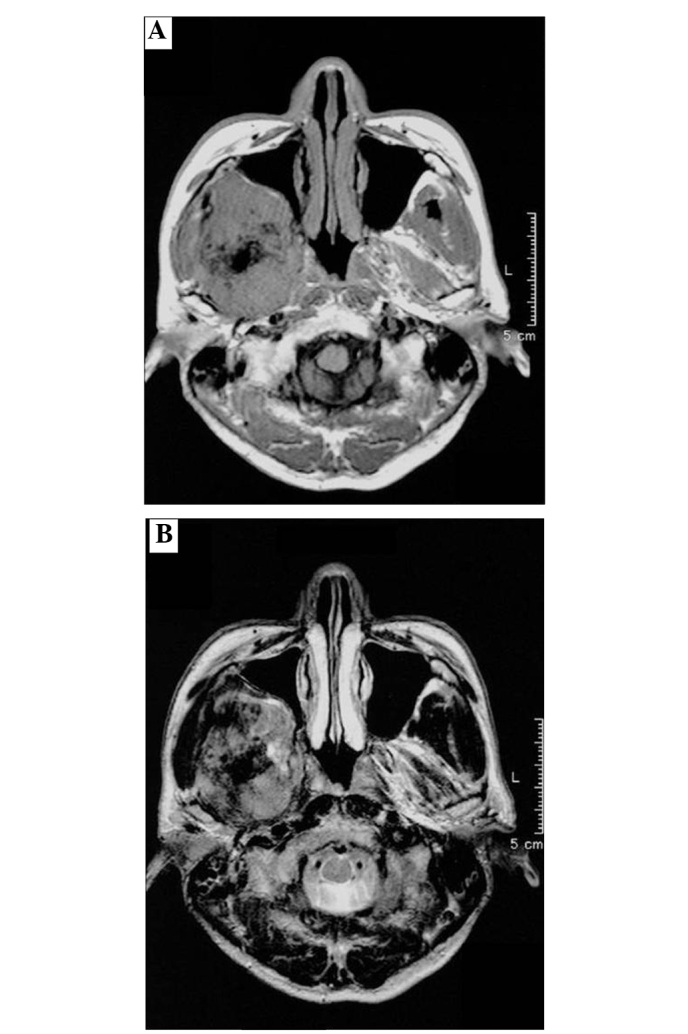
Low-intensity within an isointensity mass on an axial view of magnetic resonance imaging showing ossification in the tumor on (A) T1- and (B) T2-weighted images.
